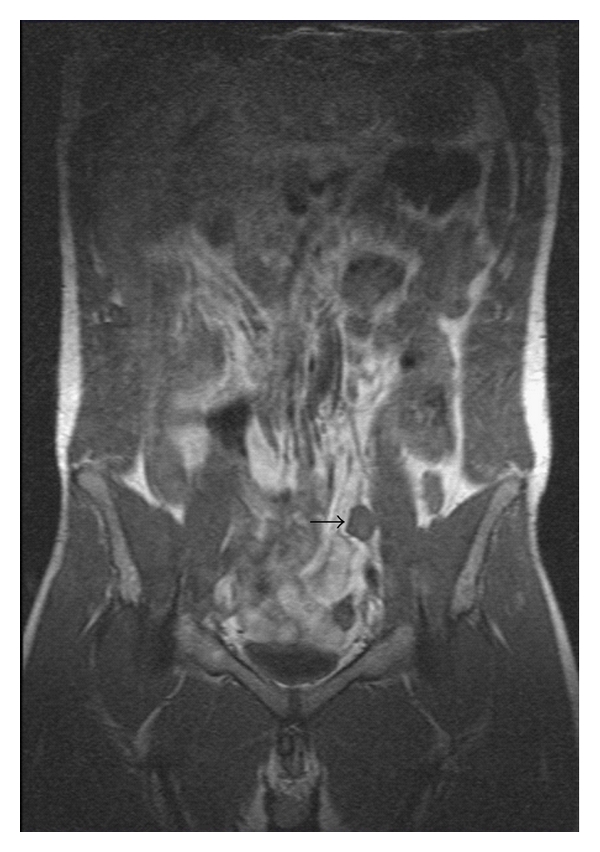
Coronal magnetic resonance imaging scan demonstrating 22 mm retroperitoneal lymph node (arrow).
